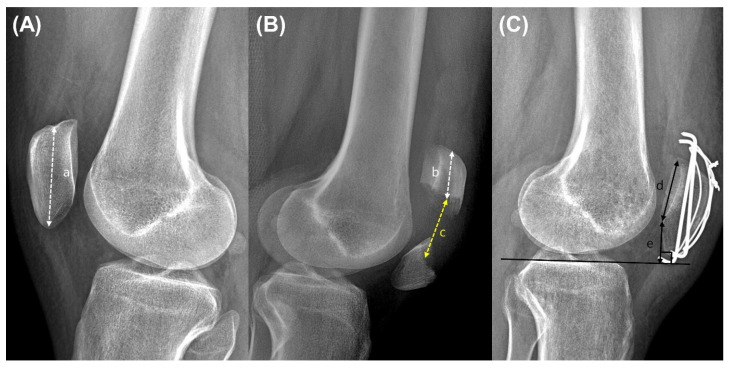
Plain radiographs of (A) contralateral side, (B) before operation, and (C) after operation. Patellar length (a) was measured from the midpoint of patellar upper pole to lower pole at contralateral side radiographs. The upper fragment length (b) was measured from the midpoint of patellar upper pole to lower end of upper fragment. The fracture gap (c) was also measured using lateral radiographs of the injured side before the operation. The fracture gap was defined as the distance between the lowest midpoint of upper fragment and the highest midpoint of lower fragment in lateral plain radiographs. The fracture location was defined as the upper fragment length divided by the patellar length (b divided by a) and expressed as a percentage. The modified Blackburne–Peel index was defined as the perpendicular distance from the lower margin of the patellar articular surface to the tibial plateau line, i.e., (e) divided by the length of the patellar articular surface (d), on lateral-view radiographs.
